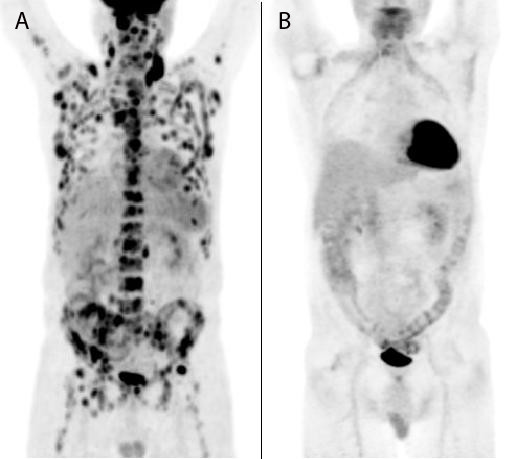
The scout film of the positron emission tomography-computed tomography (PET-CT) scan performed prior to this first round of chemotherapy (a) demonstrates diffuse regions of uptake involving multiple ribs, multiple vertebral bodies, the pelvis, the sternum and the scapula. There is also increased fluorodeoxyglucose (FDG) uptake in multiple bilateral lymph node regions extending from the jugular, supraclavicular, mediastinal, retroperitoneal, pelvic and inguinal regions, consistent with Hodgkin's lymphoma. There is increased FDG uptake in the posterior and right lateral walls of the nasopharynx. About two months after his first round of chemotherapy, a repeat PET-CT scan (b) showed a marked interval decrease in the FDG-avid metastatic burden.
